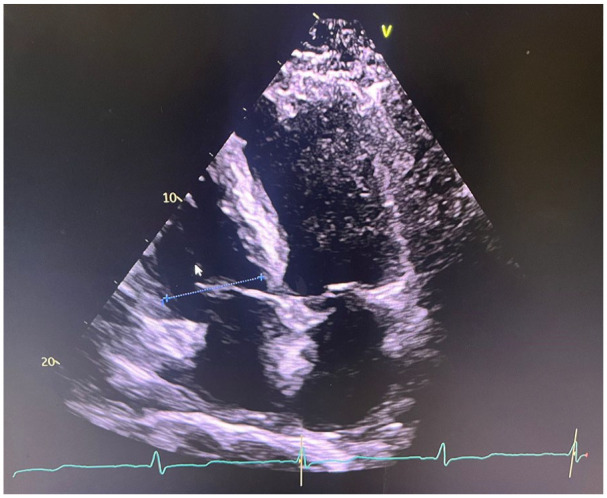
Apical 4-chamber echocardiogram view showing moderate left ventricular wall thickening with a granular, speckled myocardium.
